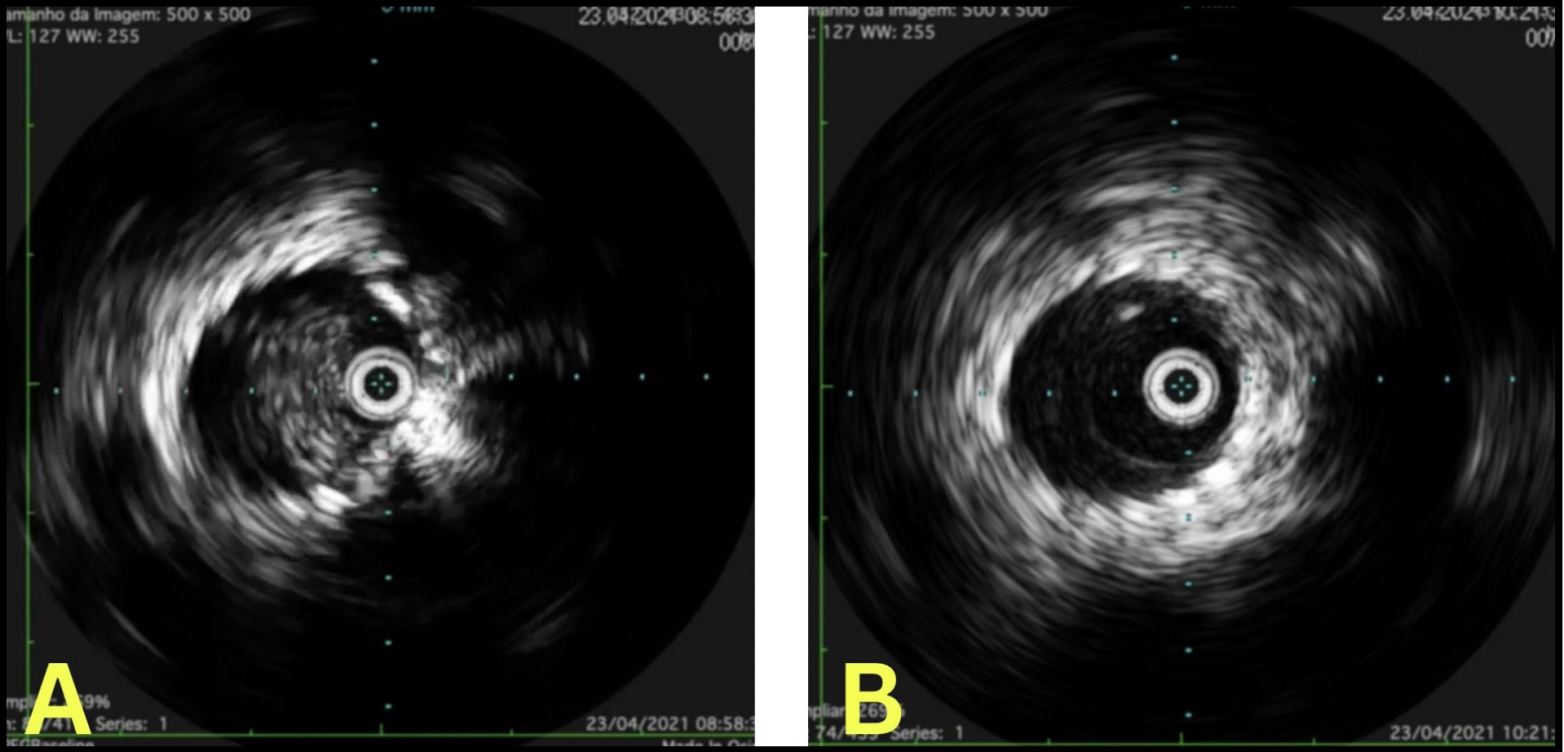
A: Intravascular ultrasound of the left common iliac vein demonstrating a previous pre-thrombectomy stent. B: Intravascular ultrasound of the left common iliac vein demonstrating free previous stent after thrombectomy.
